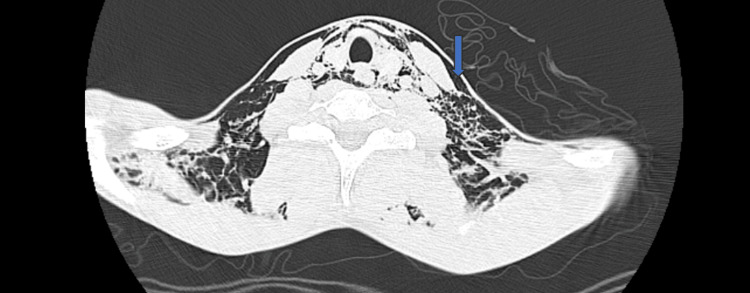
Axial chest CT image showing bilateral cervical subcutaneous emphysema. The arrow highlights air dissecting the subcutaneous tissues.
